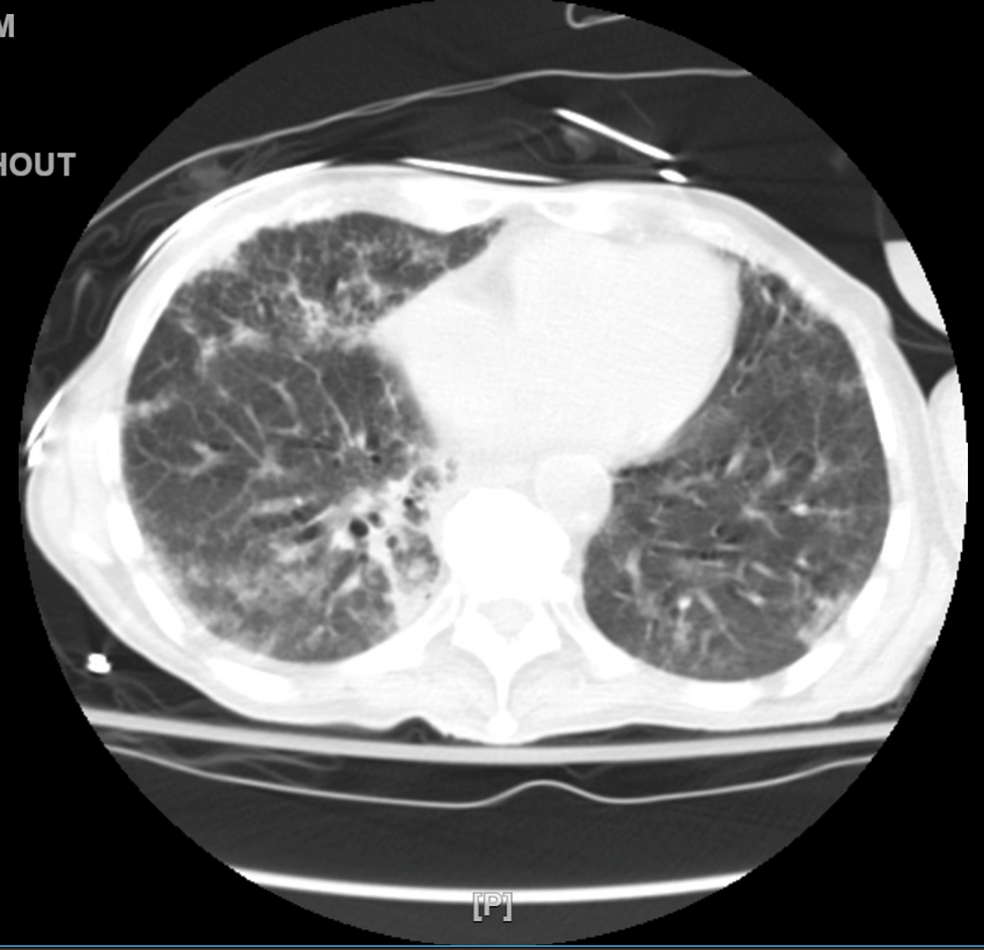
High-resolution CECT chest performed on day 1 of the hospital stay The image shows diffuse bilateral reticular ground glass opacities in the lower lung lobes. CECT: contrast-enhanced computed tomography
